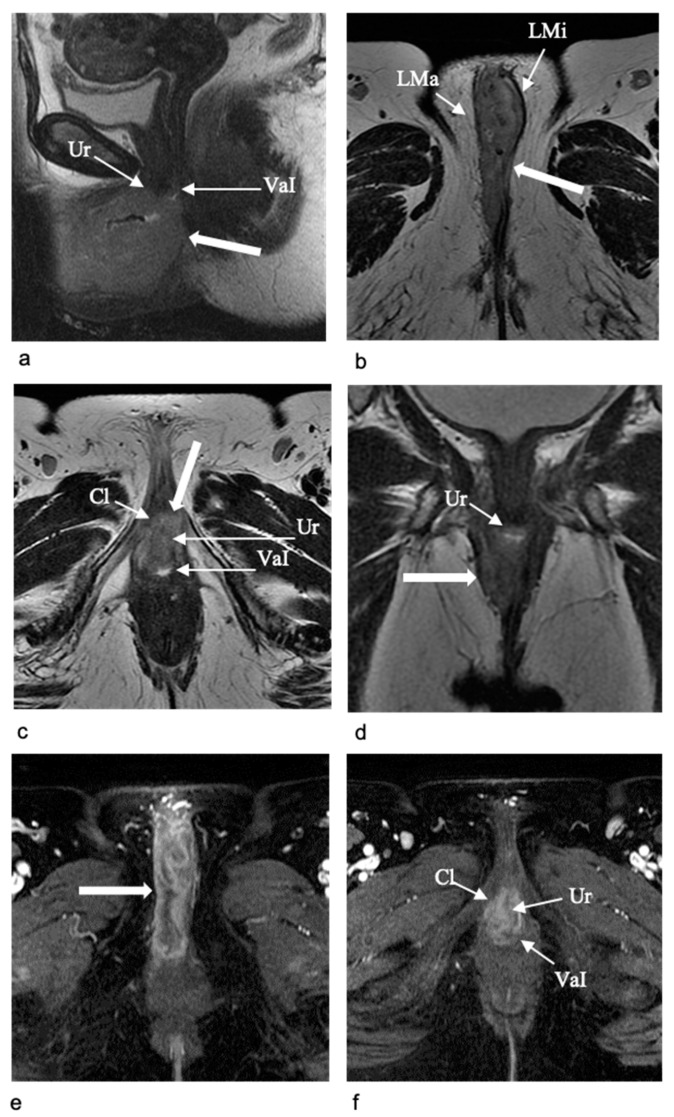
Stage II, in a 63-year-old woman with vulvar carcinoma. Sagittal (a) and axial T2-W MR images at the level of perineum (b) and of vestibule (c) show a bulky vulvar mass (arrow) involving the labia majora (LMa), labia minora (LMi), and clitoris (Cl), extending posteriorly to the urethral meatus (Ur) and vaginal introitus (VaI). On the coronal T2-W MR image (d) the involvement of the urethral meatus is also evident (Ur) by the tumor. Axial contrast-enhanced T1-W MR images at the level of perineum (e) and of vestibule (f) show the intense enhancement of the tumor and its extension to the clitoris (Cl), the urethral meatus (Ur) and the vaginal introitus (VaI) posteriorly.
